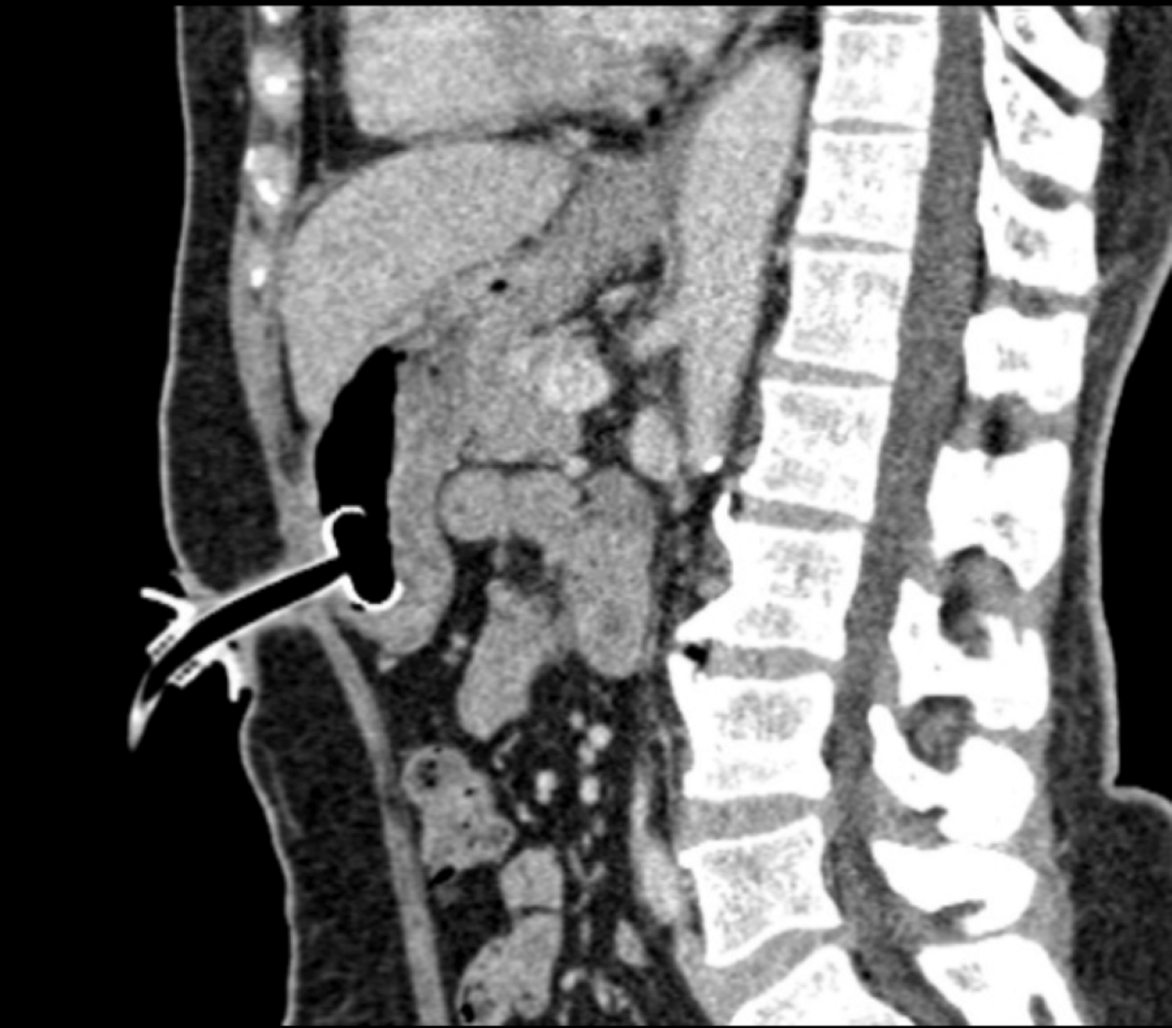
Correct Placement of a Percutaneous Gastrostomy Tube Sagittal non-contrast CT image demonstrating appropriate positioning of a percutaneous gastrostomy tube. The inflated retention balloon is located within the gastric body (arrow), confirming intragastric placement. Recognition of this appearance is essential to differentiate correct positioning from perigastric or intraperitoneal malposition.
